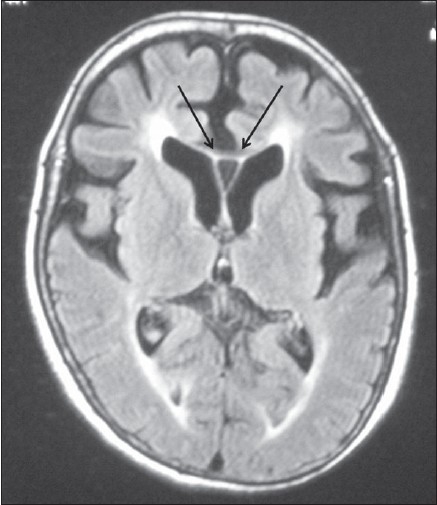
Axial FLAIR image showing white matter lesions with frontoparietal lobe atrophy with thin corpus callosum (arrows) and prominent lateral ventricle with cavum septum pellucidum
